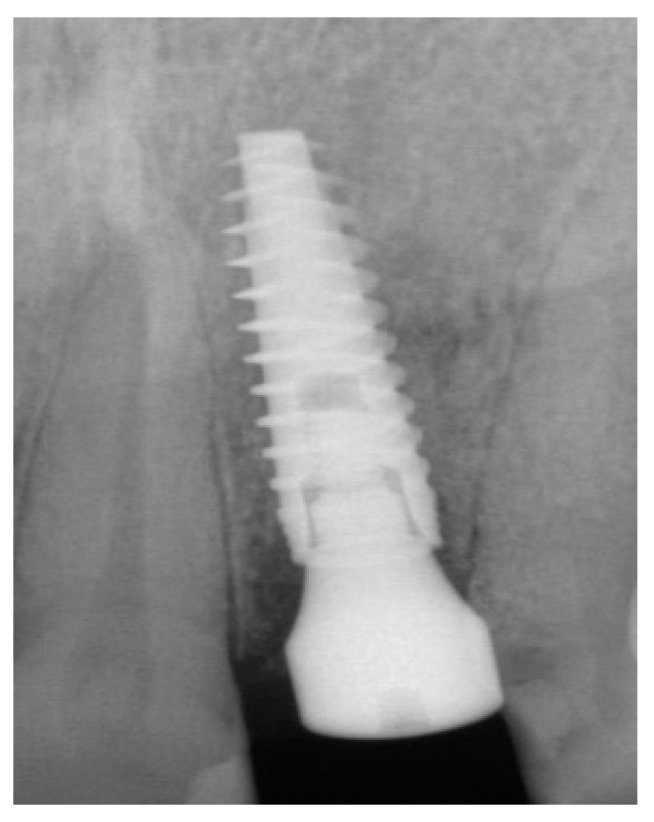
Radiograph of the lateral incisor.
